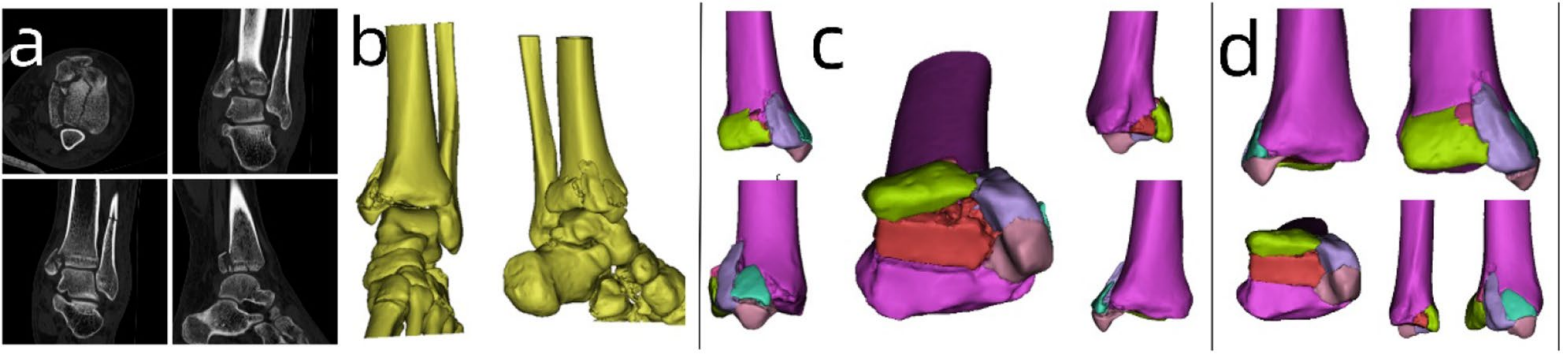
(a) CT imaging data of the fractured area. (b) Three-dimensional modeling of the fracture data using Mimics software. (c) Isolation of fracture fragments and color-coded annotation using the threshold circle selection function. (d) Virtual anatomical reduction of the fracture fragments using the move-rotate function.
